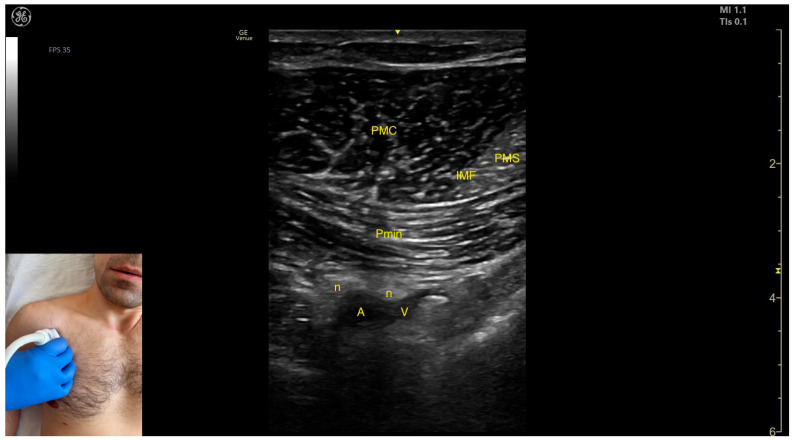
Ultrasound anatomy of the pectoralis major (Pmaj) with key landmarks: PMC—pectoralis major clavicular head; PMS—pectoralis major sternocostal head; IMF—intramuscular fascia; Pmin—pectoralis minor; n—nerve; A—artery; and V—vein.
